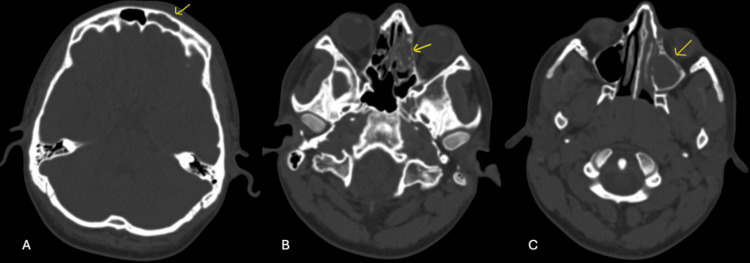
Computed tomography of the head in axial view and bone window. A: Left frontal sinus occupied (arrow). B: Left ethmoidal cells occupied (arrow). C: Left maxillary sinus occupied (arrow).
